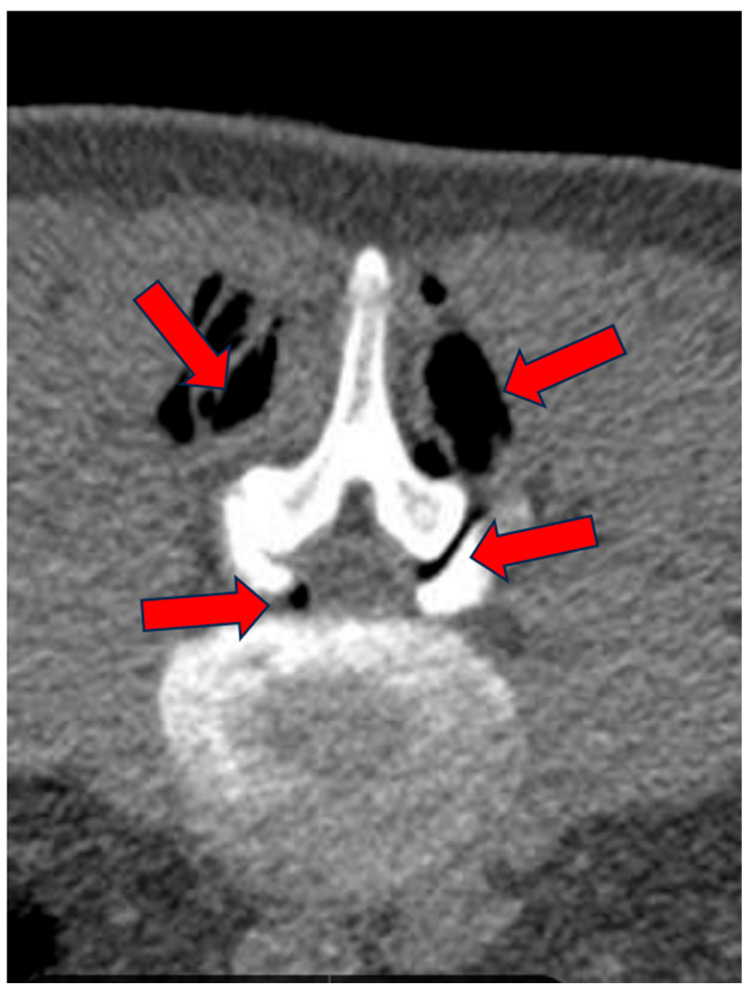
Low-dose CT scan for proper distribution of the gas mixture after infiltration (arrows).
